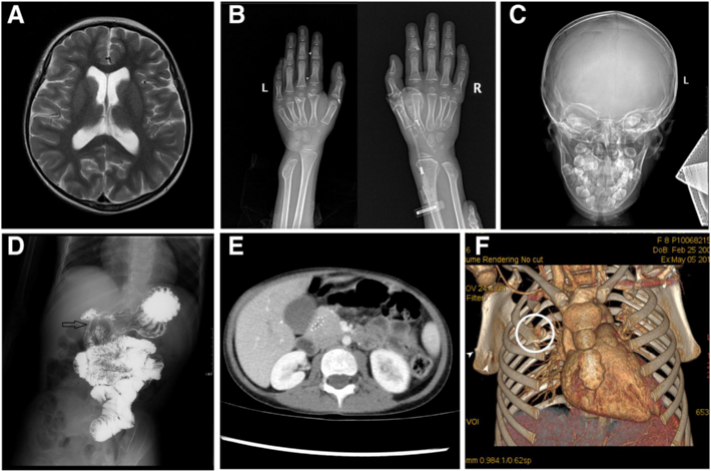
Imaging examinations of the patient. a Brain MRI showed encephalatrophy-like changes. b, c X-ray examination showed short metacarpals of the fifth finger, cone-shaped epiphyses of the proximal inter-phalangeal joint of middle phalanx of the fingers (white arrow), multiple exostoses (white arrow head) and malaligned crowded teeth. d Upper GI series showed insufficient distention at the pylorus and duodenum (black arrow) indicating encircled pancreatic tissue. e Axial CT image clearly showed annular pancreatic tissue encircling the second portion of the duodenum (white circle). f CT volume rendering revealed exostoses (white arrow head) and fusion malformation of the second and third ribs on the right side (white circle)
